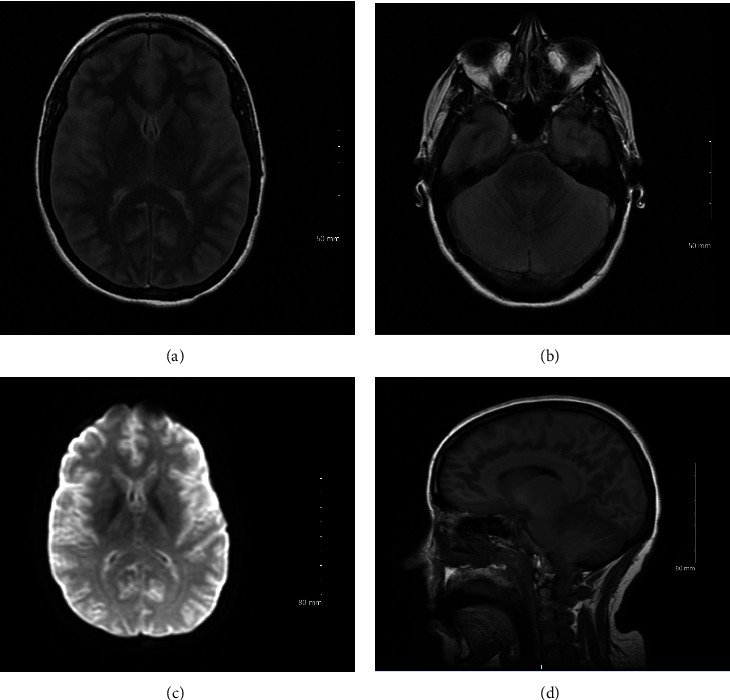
(a) MRI brain, axial T2 FLAIR, at the level of the ganglionic layer. (b) MRI brain, axial T2 FLAIR, at the level of the 4th ventricle. (c) MRI brain, axial DWI sequence. (d) MRI brain, sagittal T1 sequence.
